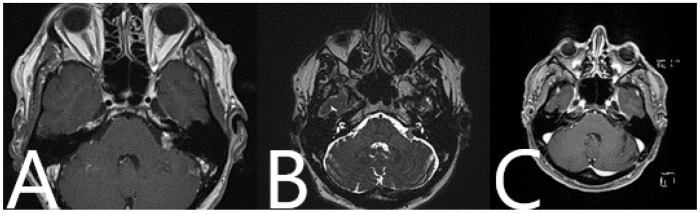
Patient No. 36. Preoperative (A) T1-weighted post-contrast and (B) T2-weighted MRI which shows a left-sided AN with contact to brain stem. (C) Postoperative T1-weighted post-contrast MRI shows complete resection of the tumor.
